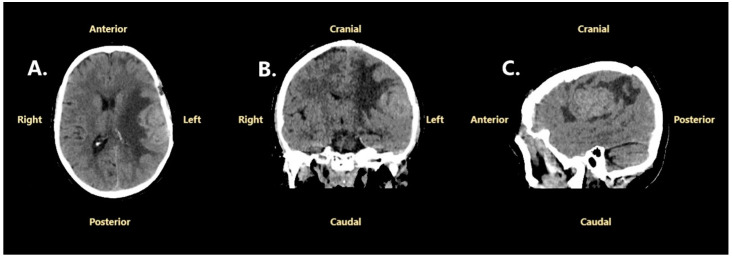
Native cranial CT scan performed in August 2024: (A)—axial projection; (B)—coronal projection; (C)—sagittal projection.
